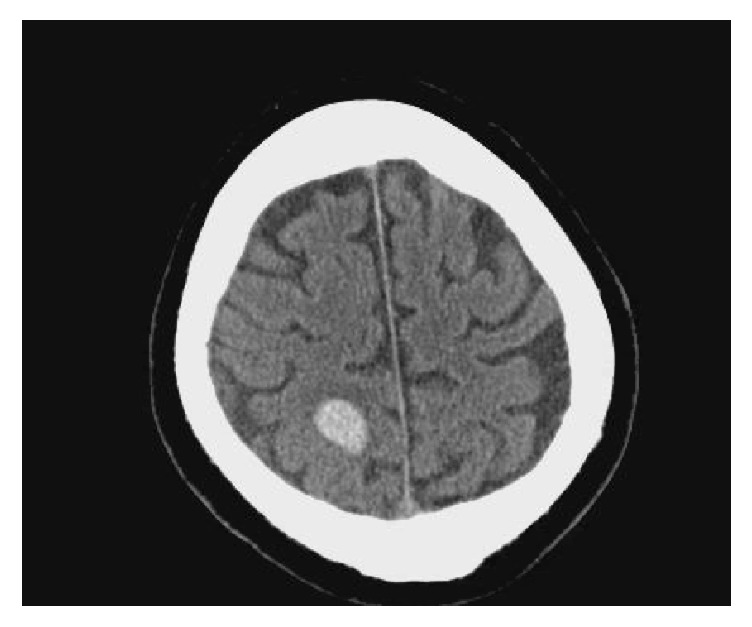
CT scan of the brain without contrast showing an acute 2.2 cm intraparenchymal hemorrhage with vasogenic edema in the posterior right parietal lobe.
